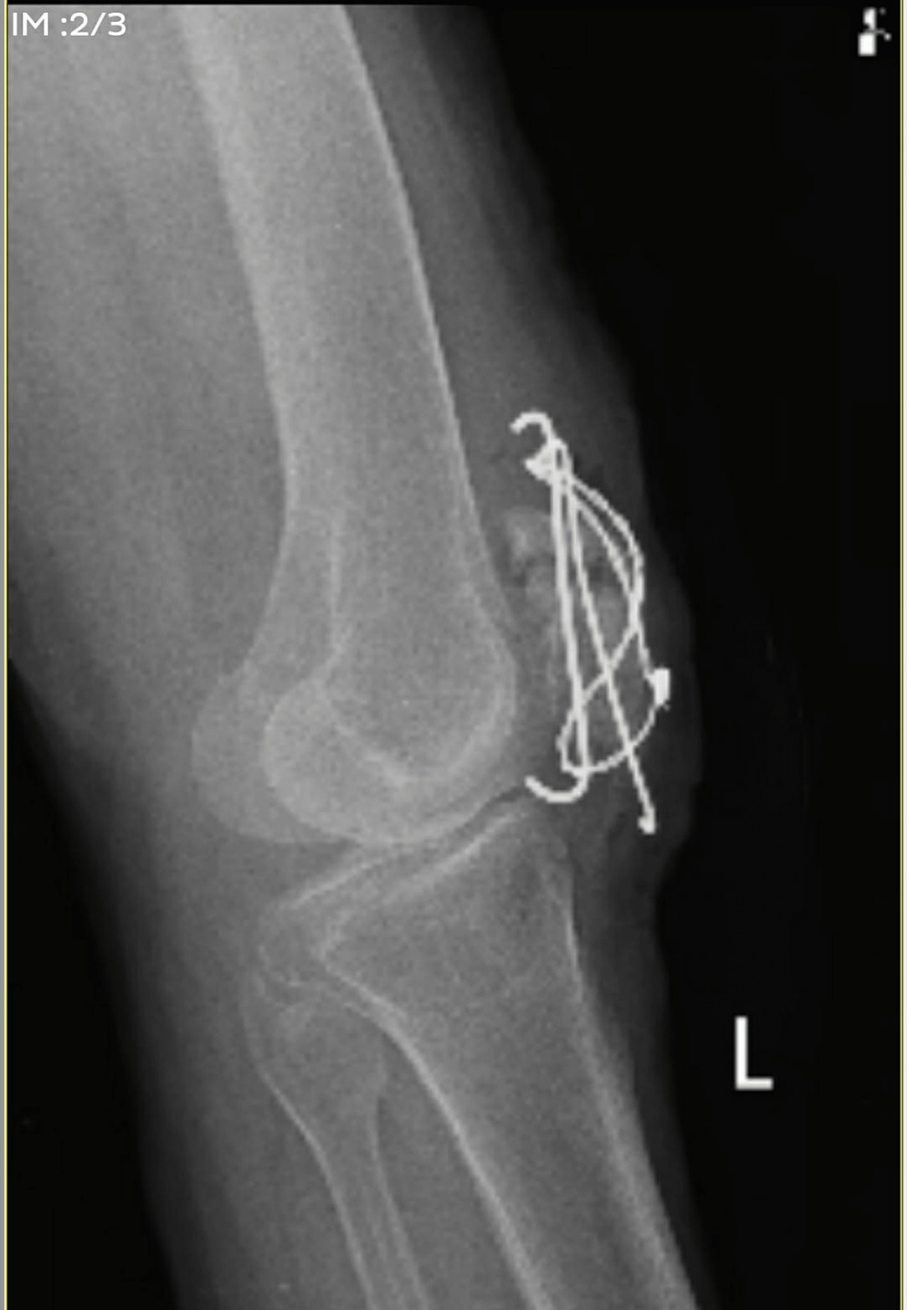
Postoperative X-ray of comminuted patellar fracture surgically treated with tension band wiring.
